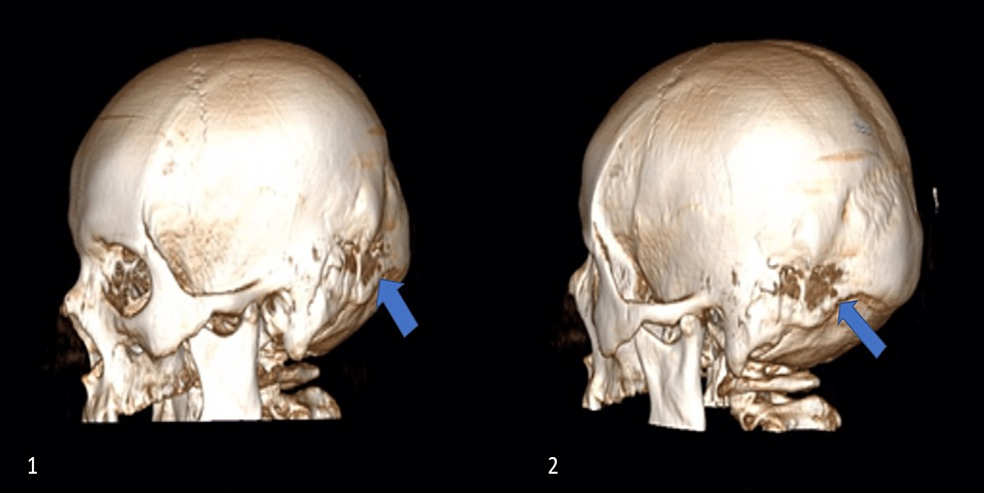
Preoperative three-dimensional reconstructed CT scan showing the left occipital bone defect (Blue arrows).
